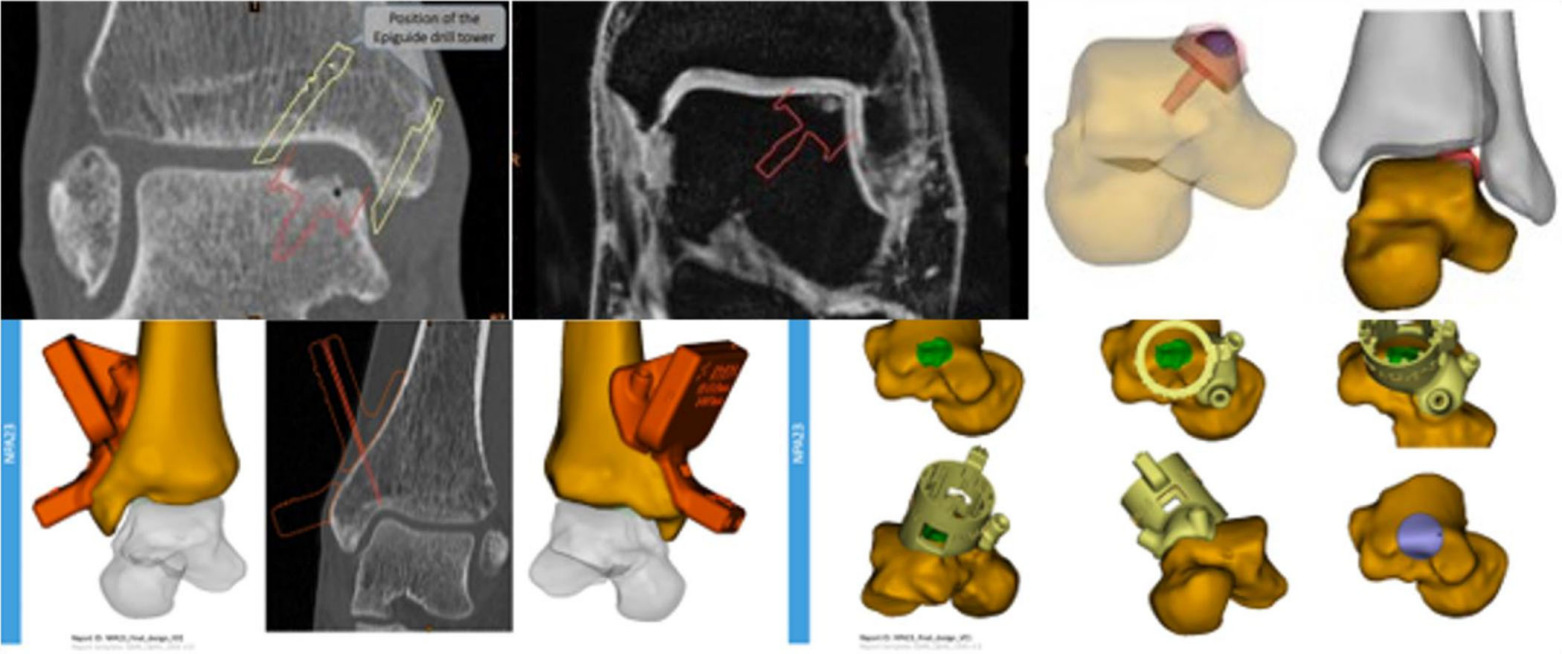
The Damage Marking Report based on computed tomography/magnetic resonance imaging scans (above) and the final design of the Damage Marking Report (below).
